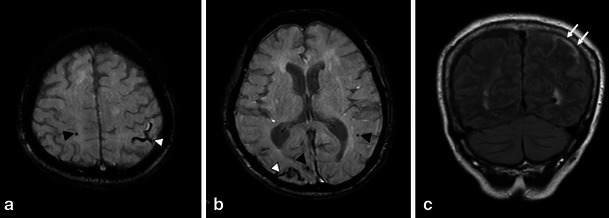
Non-traumatic SAH at the convexity of the brain, microbleed and SS in a 72-year-old woman who was clinically diagnosed with AD. Axial 3D T2*-weighted images (a, b) showed bilateral subcortical MBs (*black arrowheads*) and sulcal SS (*white arrowheads*) in the posterior dominant distribution. Additionally, SAH along the left parietal sulci (*arrows*) was identified on a coronal FLAIR image (c)
